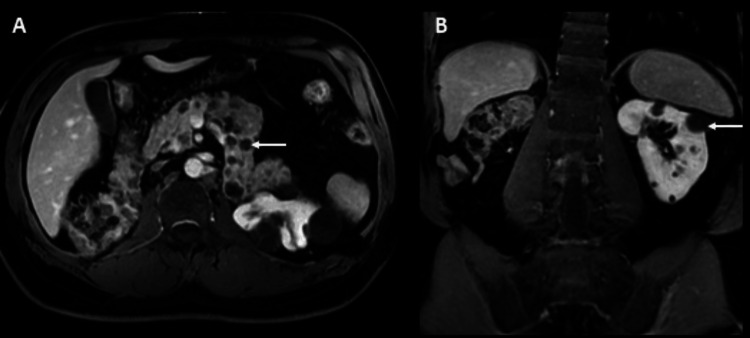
Abdominal magnetic resonance imaging (axial and coronal views). Axial (A) and sagittal T1WI fat-saturated with gadolinium show multiple pancreatic (A) and renal (B) cysts (arrows).
